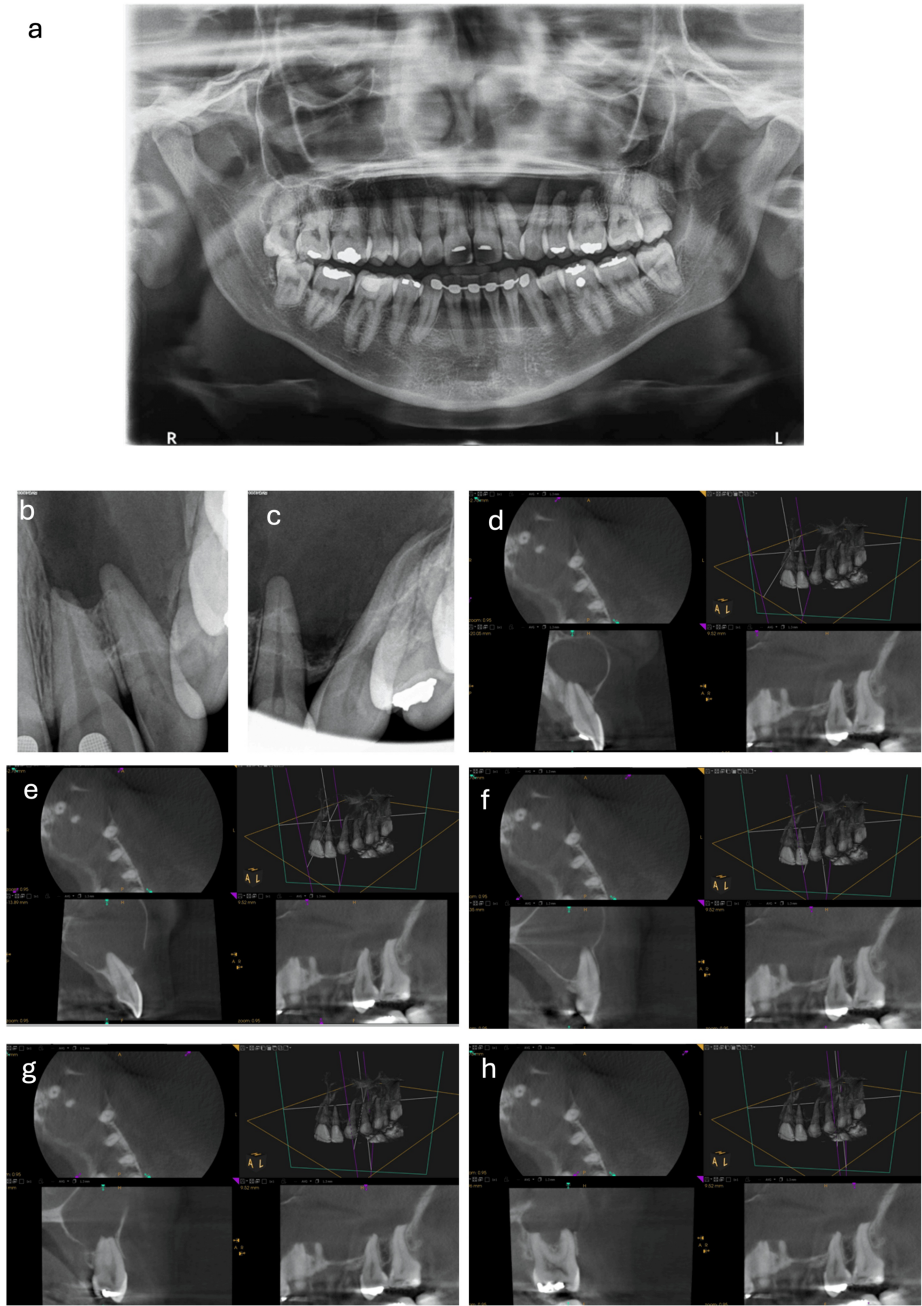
Case scenario A 35-year-old female patient (ASA I) came to the dental clinic complaining of pain and swelling. The pain, during biting, and the swelling are related to the upper left central maxillary incisor extending to the upper left first molar. Her medical & social histories are insignificant. She had a history of trauma. Clinical examination revealed: an eggshell crackling swelling covered with normal mucosa. Aspiration resulted in clear cystic fluid. Radiographic examination showed: A well-defined radiolucent lesion surrounded with a radiopaque line involving teeth from #21 to #26. Teeth from #23 to #26 are within normal limits (WNL). Tooth #21 responded negative to cold and electric pulp test (EPT) with mild pain on percussion. Tooth #22 responded with lingering pain to cold test and normal response to percussion. (a) Panoramic radiograph; (b,c) pre-operative periapical radiographs; (d) Cone beam computed tomography (CBCT) image of tooth #21; (e) CBCT of tooth #22; (f) CBCT of tooth #23; (g) CBCT of tooth #25; (h) CBCT of tooth #26.
